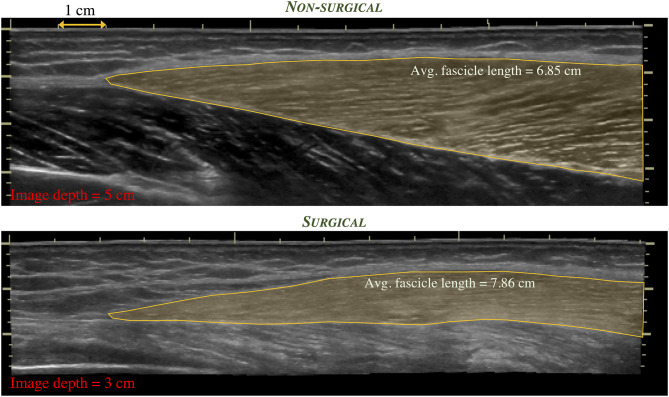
Extended field-of-view ultrasound images depicting the distal region of the vastus lateralis muscle in the same individual as in Figure 1. Differences in regional muscle thickness, fascicle orientation, and length (shaded region) can be appreciated between the non-surgical limb (top) and the surgical limb (bottom).
